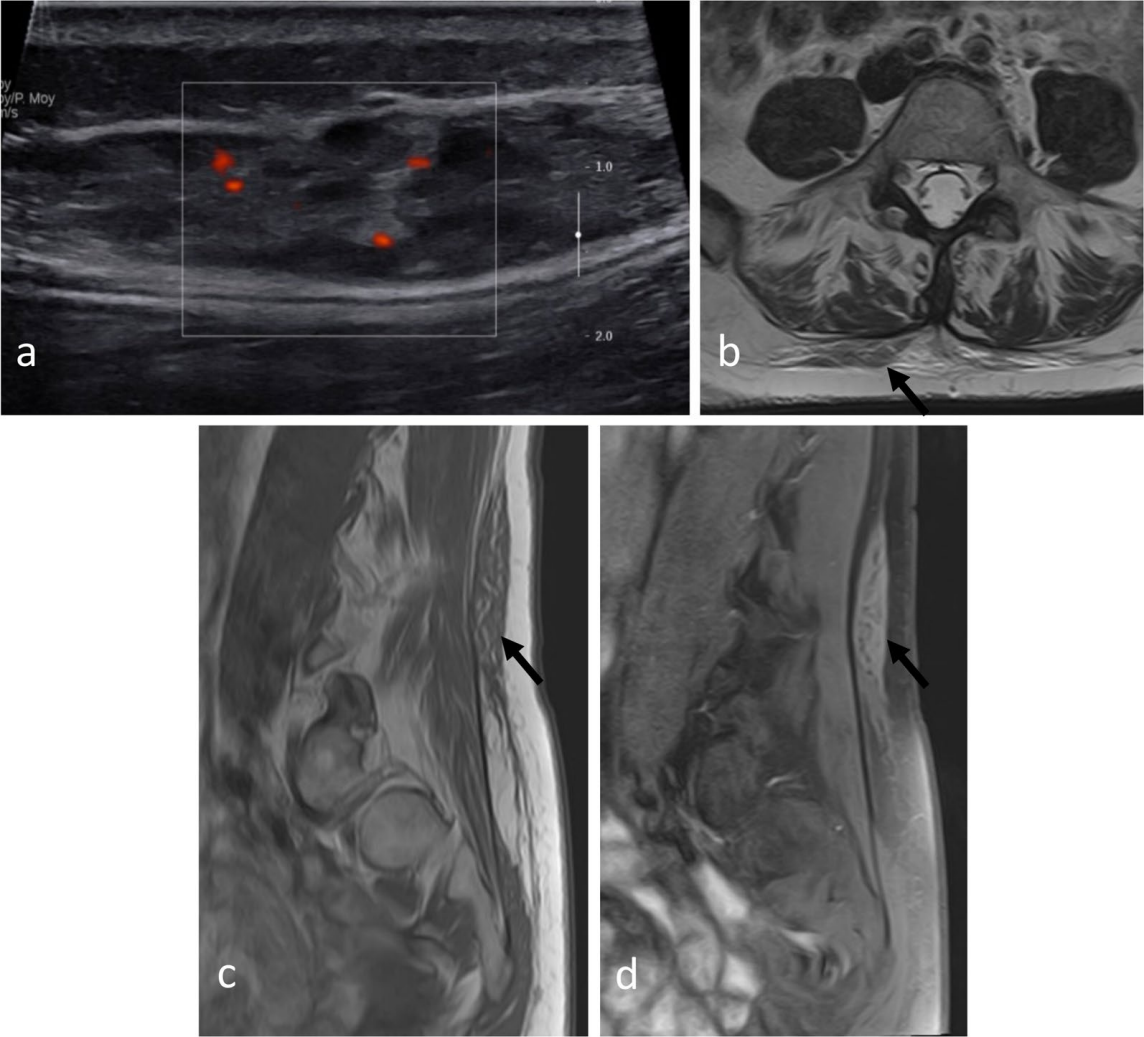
Adiponecrosis* in a 43-year-old male with low back pain 2 months after a fall. US (a) demonstrating a hyperechoic, superficial mass and power Doppler signal showing hypervascularity. Axial T2WI (b) and sagittal T1WI (c) showing a superficial mass (arrows) with heterogeneous iso- to hyperintense signal. Sagittal contrast-enhanced fat-suppressed T1WI (d) showing a heterogeneous enhancement
